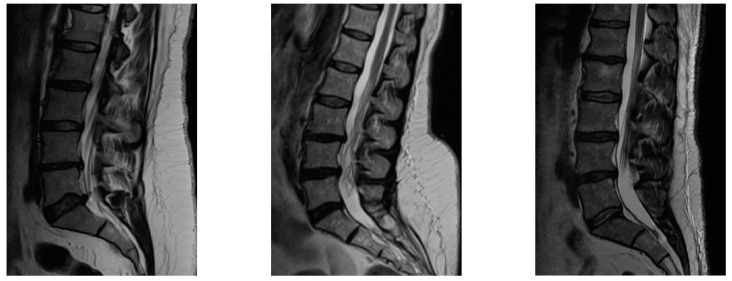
Sample MRI imaging of treated hernias/protrusions.
